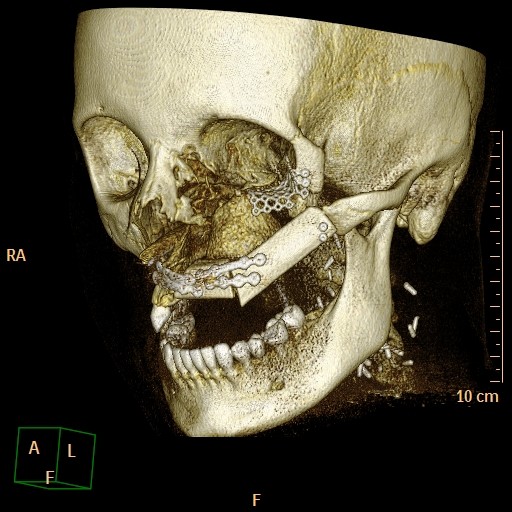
Postoperativ CT 3D reconstruction.
